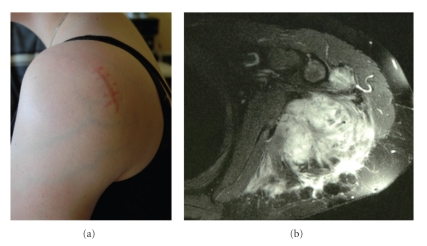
Clinical findings at diagnosis. Note the bulky mass of the shoulder with collateral circulation (a). MRI (T1 gadolinium sequences) shows a heterogeneous tumor infiltrating muscles and subcutaneous tissue (b).
